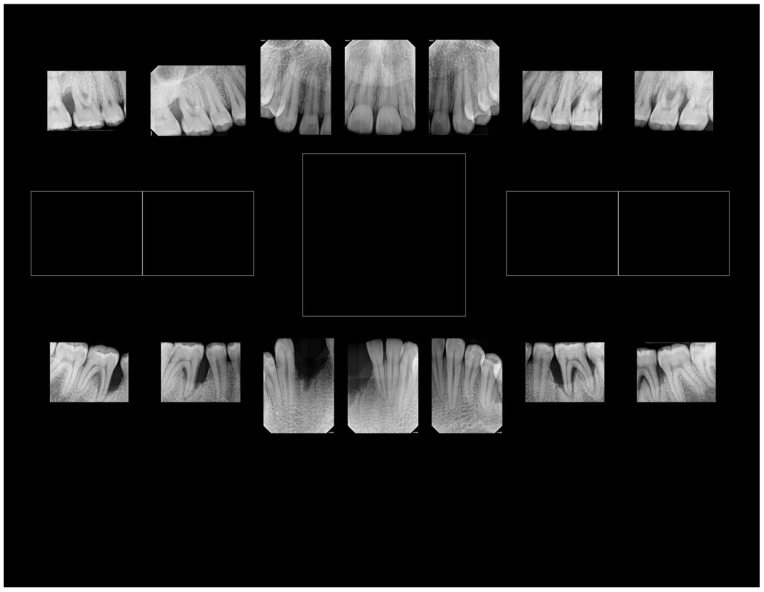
Radiographs of a patient with significant pocket depth and bone loss. Shows loss of two mandibular incisors and extensive bone loss in the first molar region,. Note the lack of carious lesions on radiographs throughout the dentition.
